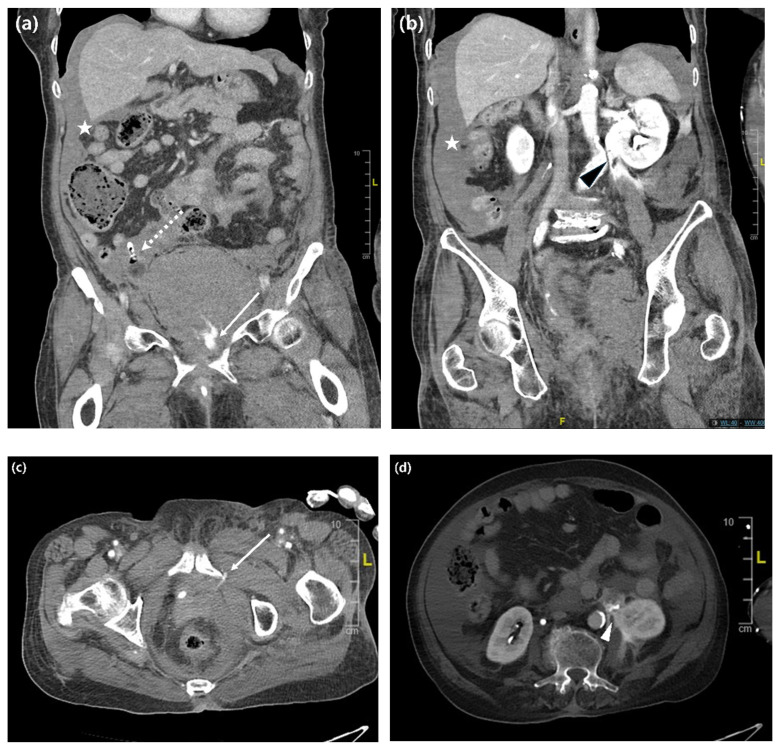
Initial enhanced computed tomography findings after the transobturator tape procedure. Coronal view of delayed phase, (a); coronal view of portal phase, (b); axial view of portal phase, (c); axial view of portal phase, contrast enhanced, (d). White asterisk: hemoperitoneum; white dotted arrow: Foley catheter; white solid arrow: active contrast extravasation from the left obturator artery; black arrowhead and white arrowhead: contrast extravasation at the left renal pelvis/ureteropelvic junction (UPJ) level.
